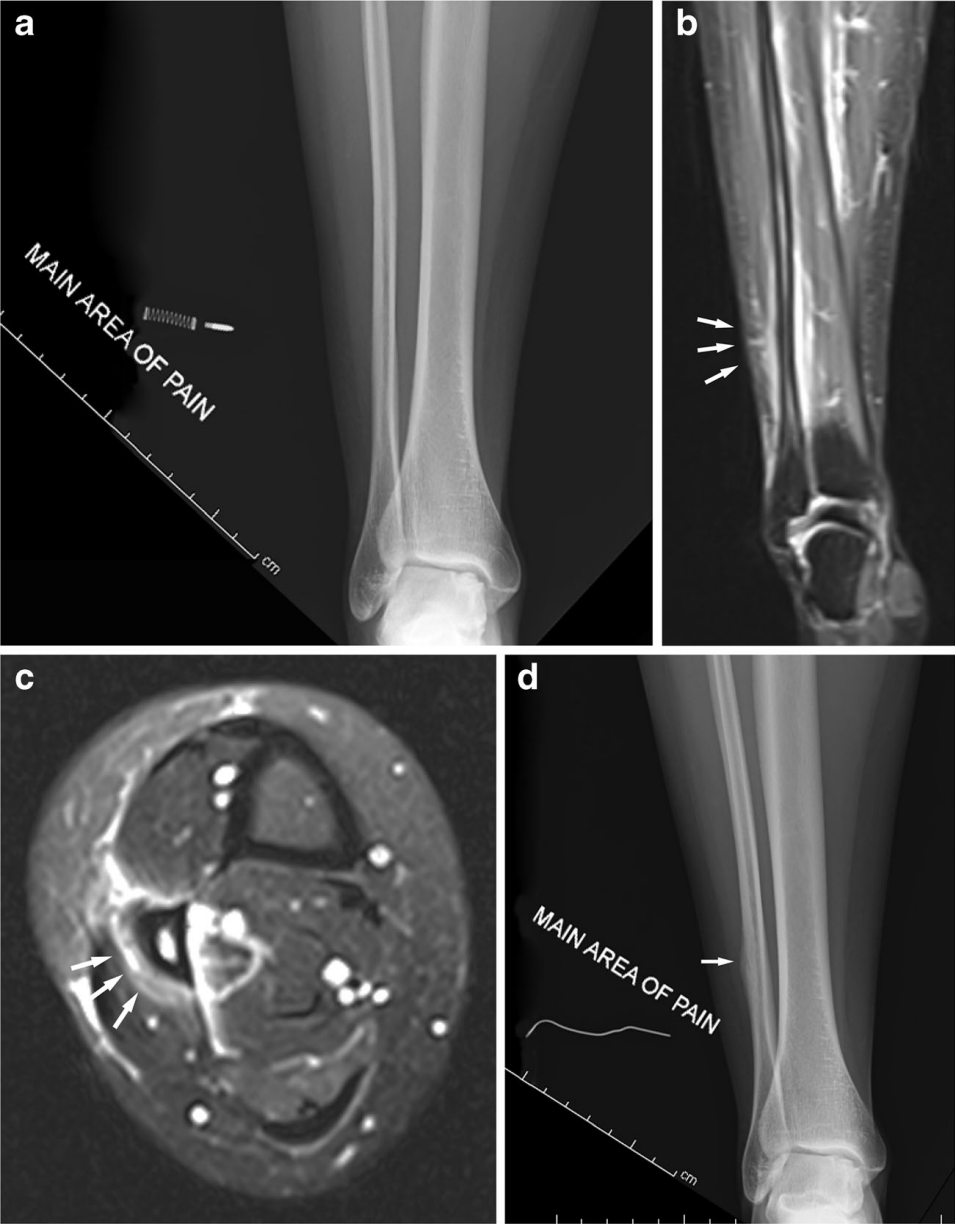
Middle-aged female runner presenting with focal calf pain. Initial radiographs (a, AP view shown) were normal. MRI 2 weeks after the initial radiographs (b, c, coronal and axial PD-FS images) shows a periosteal reaction and widespread soft tissue oedema around the fibula shaft typical for a stress fracture (see arrows). Radiographic follow-up 3 weeks after this (d) shows a periosteal reaction of the fibula where pain was initially indicated (arrow). Radiographs are insensitive for stress fractures early on; MRI shows soft tissue oedema and a periosteal reaction and is the investigation of choice if radiographs are negative
